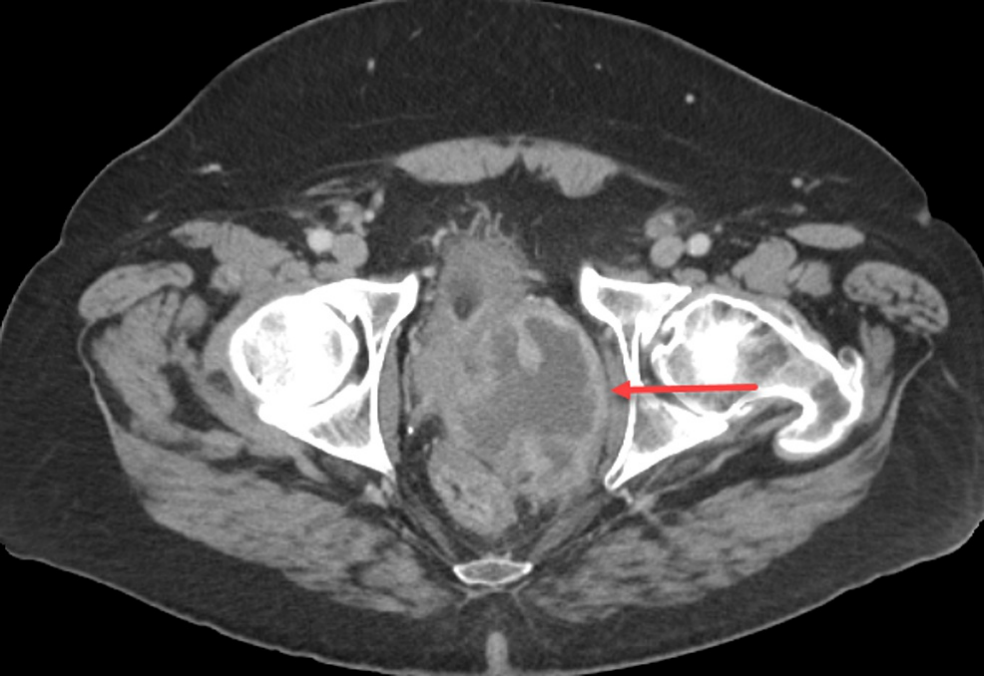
Axial CT pelvis showing enlarged prostate (9.6cm) (red arrow) with a mass effect on the rectum and bladder
